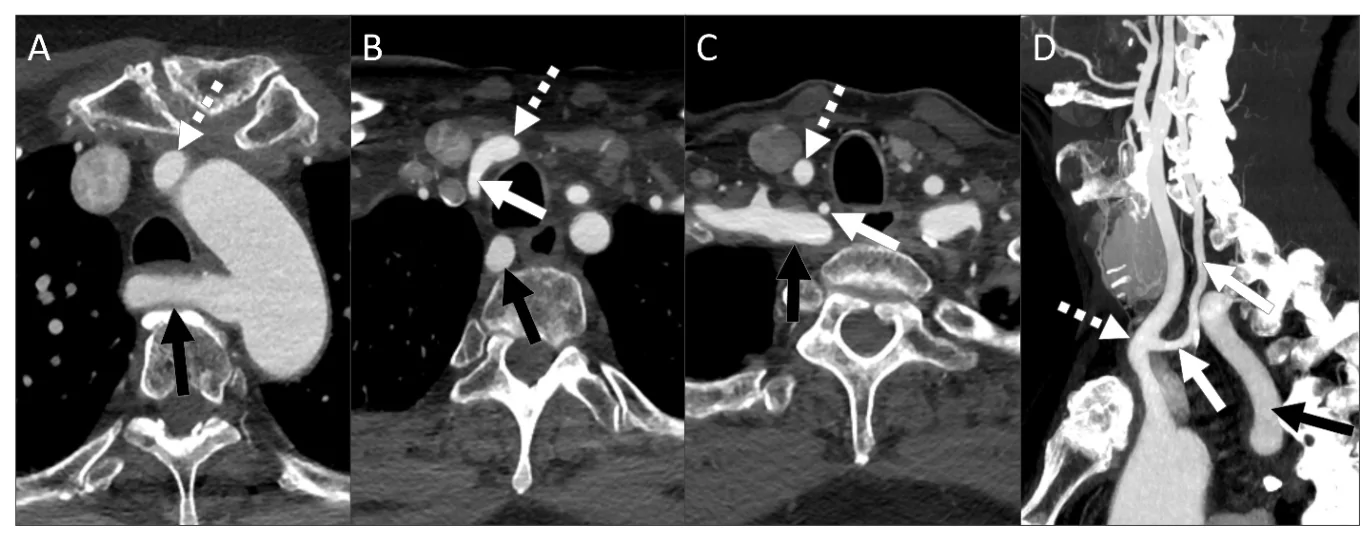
Right VA originating from the right CCA. Axial (A–C) and oblique sagittal MIP (D) CTA images demonstrate an aberrant right subclavian artery (black arrows) originating from the distal aortic arch and an aberrant origin of the right VA (white arrows) from the proximal right CCA (dashed white arrows).
